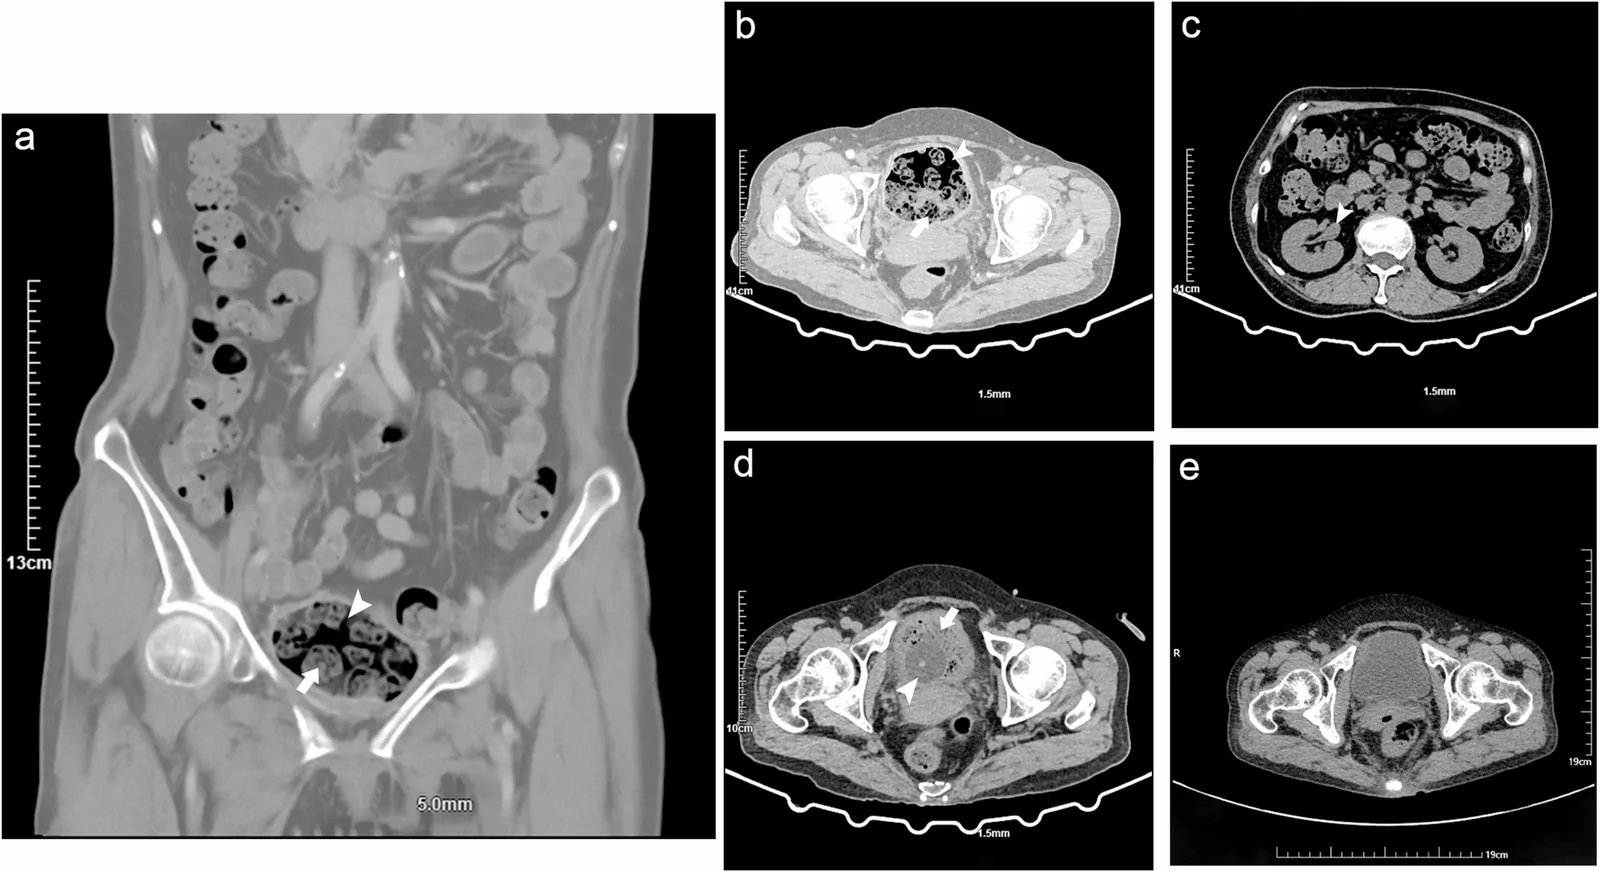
(a) Coronal view of abdominal CT demonstrating intraluminal gas (arrow head), multiples of isolated round masses (arrow) and bowel gas. (b) Axial contrast-enhanced abdominal CT scan (venous phase) showing a distended and clear outline bladder with diffuse wall thickening, irregular intraluminal gas (arrow), and intraluminal masses (arrowhead) similar to those described in a. (c) Mild right hydronephrosis. (d) Axial non-contrast abdominal CT scan after 1 week of antifungal therapy and bladder irrigation showing coalescent nodules (arrow) and the Foley catheter balloon (arrow head) within the bladder, with resolution of the previously observed gas. (e) Two weeks follow-up after discharge, pelvic CT demonstrated regression of the intramural gas and bladder mass
